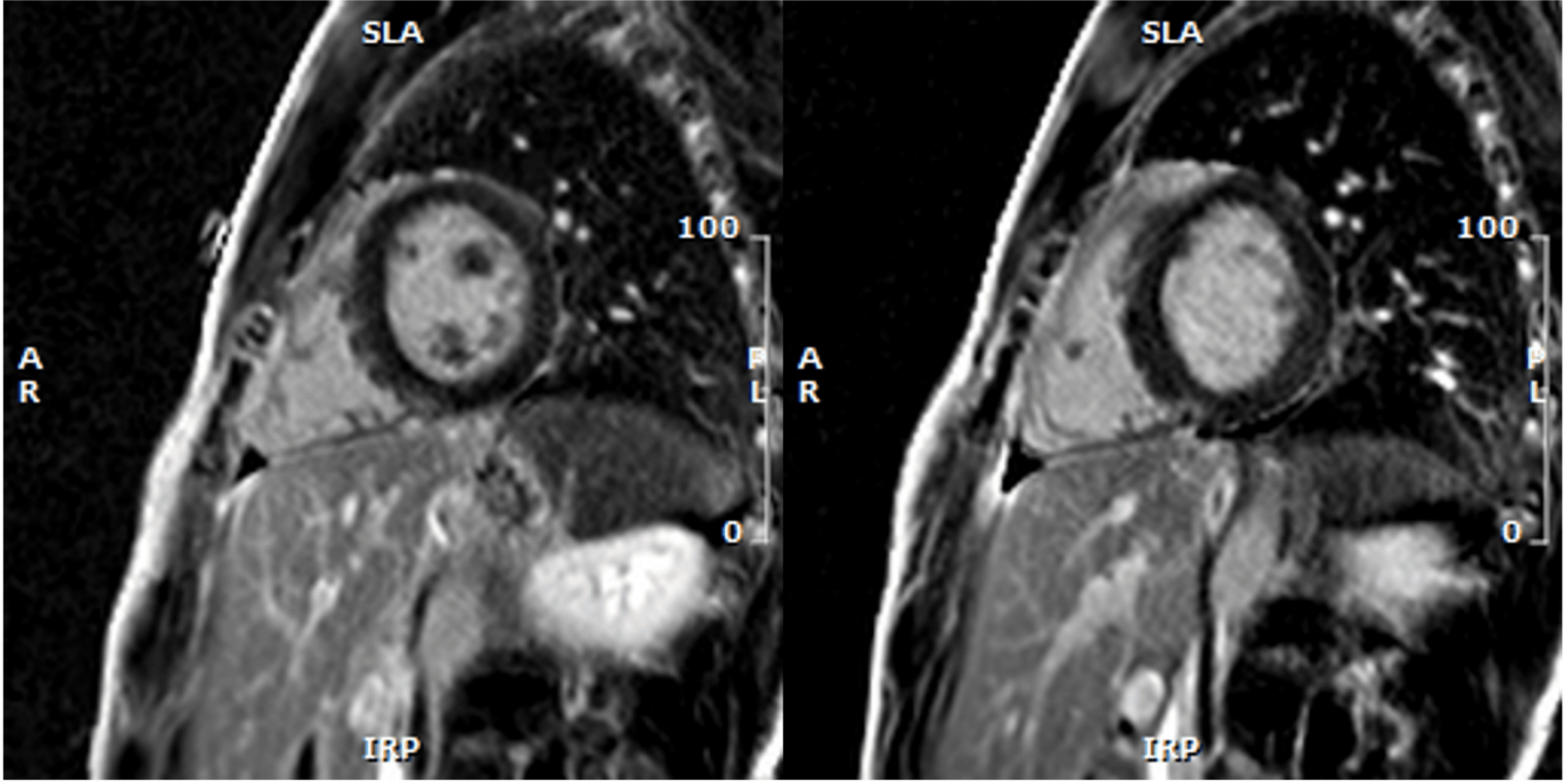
Cardiac MRI with gadolinium enhancement, demonstrating mild late enhancement at the inferior insertion point of the right ventricle into the septum. The elevated native T1 times and extracellular volume (ECV) values may indicate hyperemia and necrosis.
